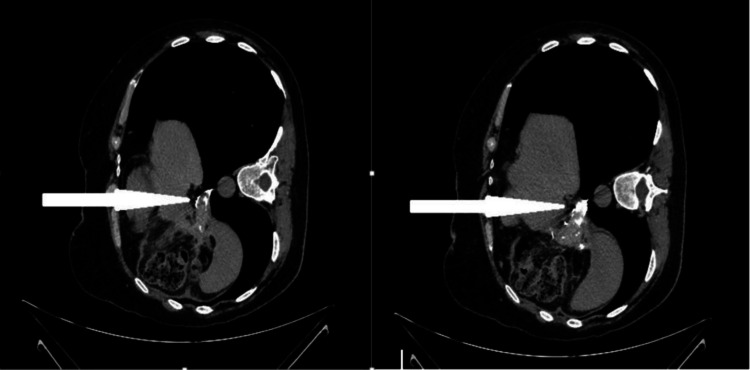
Abdominal CT scan images (axial views with the patient in left lateral position) showing complete resolution of the left subdiaphragmatic collection with no obvious contrast leak from the gastric tube (the white arrows).
